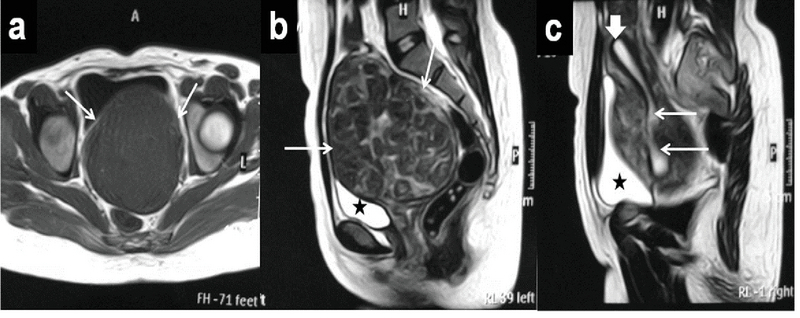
Preoperative MRI of a huge anterior cervical myoma occupying the pelvic cavity in a 27-year-old nulligravida woman, a. Axial T1-weighted MR image shows a low SI of the mass (white arrows), b. Sagittal T2-weighted MR image shows a mixed low and intermediate SI of the mass (white arrows), c. Right parasagittal T2-weighted MR image shows a uterine body on the top of the mass (thick white arrow) with an elongated cervical canal in the posterior aspect of the cervical myoma (thin white arrows), MRI: Magnetic resonance imaging; SI: Signal intensity.
